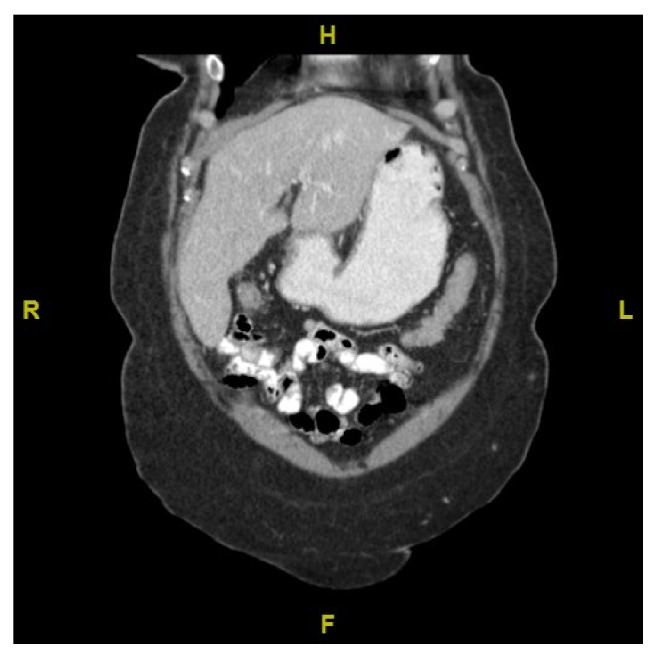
Follow-up CT sagittal view of abdomen at 7 weeks indicates marked improvement.
